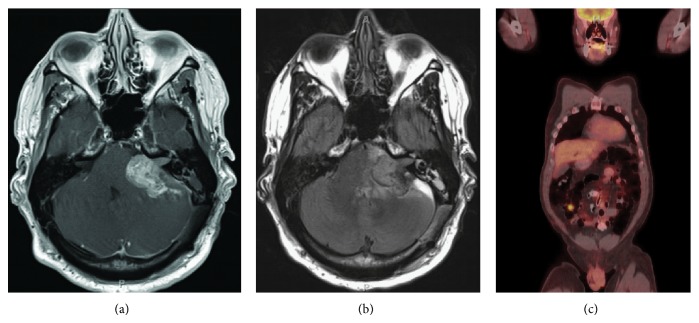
(a) T1-axial MRI with contrast and (b) T2-axial Flair MRI demonstrating enhancing mass in the left cerebellopontine angle 8 weeks later. (c) Post-operative whole body positron emission tomography demonstrating increased uptake in the right lower quadrant corresponding to the small intestine.
